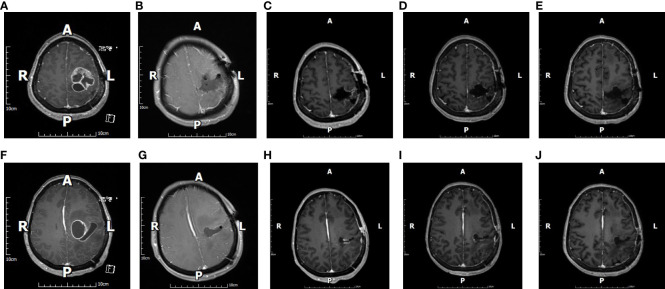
The figure shows images of a 45-year-old female patient with glioblastoma. Preoperative cranial magnetic resonance imaging (MRI)-enhanced images (A, F) suggest that the tumor was located in the left parietal lobe, and images taken at the same sections 48 h after surgery (B, G) show gross total resection (GTR). Cranial MRI-enhanced images were reviewed in the same sections 1 month after surgery (C, H), 2 months after surgery (D, I), and 6 months after surgery (E, J). All images were suggestive of GTR.
